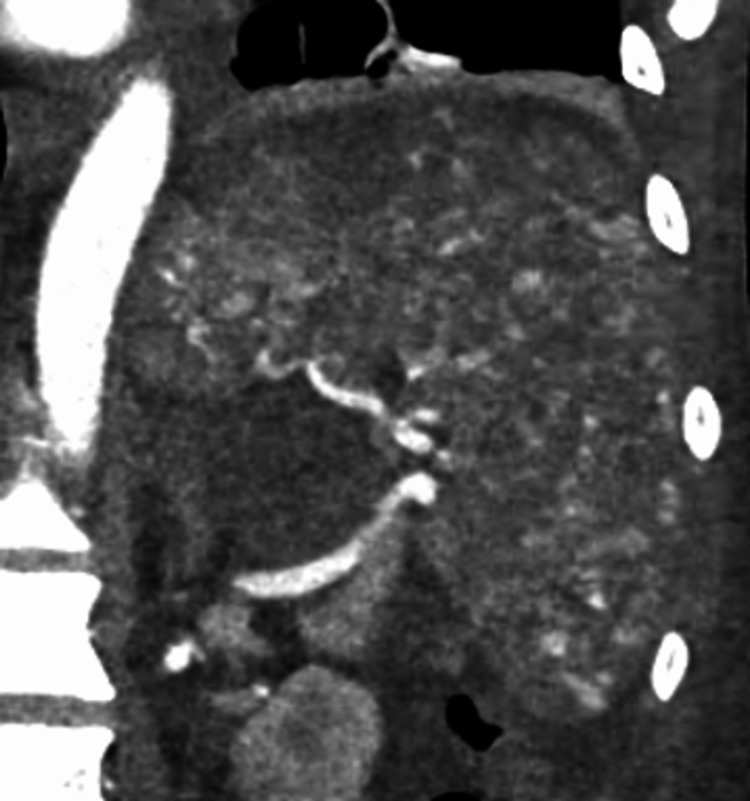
Innumerable small punctate foci of intraparenchymal contrast enhancement in a traumatic spleen on coronal CT
